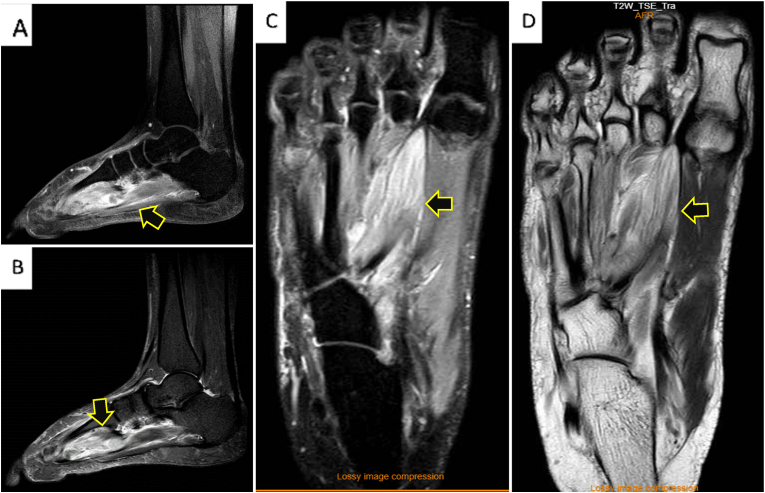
(A): is a Magnetic resonance imaging (MRI) of right foot (RF) with T1-weighting, (B): is an MRI of RF with Short Tau Inversion Recovery (STIR) sequence, (C): is an MRI of RF with Proton density (PD) weighting, and (D): is an MRI of RF with T2-weighting, (Arrows define the significant findings).
